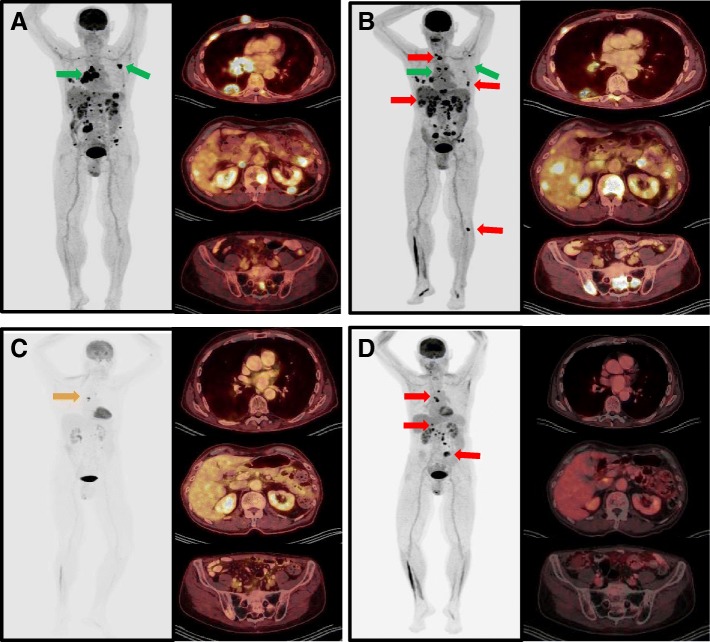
Positron emission tomography (PET) maximum intensity projection (MIP) images and axial images from the chest, abdomen, and pelvis demonstrating the radiographic response to pembrolizumab therapy. a Baseline imaging shows extensive metastatic disease burden, and green arrows highlight lesions first to improve in lung, mediastinum, and axillary lymph nodes. b Imaging after 3 months (4 cycles) of pembrolizumab are consistent with mixed response and pseudoprogression, with several new hypermetabolic foci (red arrows) in soft tissue, liver and bone. c Imaging studies at 6 months shows a near complete response to 7 cycles of pembrolizumab, with regression of multiple hypermetabolic metastatic foci, and few small remaining foci of residual hypermetabolism in the chest and abdomen. d Imaging at 12 months shows persistent FDG avidity in mediastinal and abdominal lymph nodes and new FDG avid lesions in liver and small bowel (red arrows)
